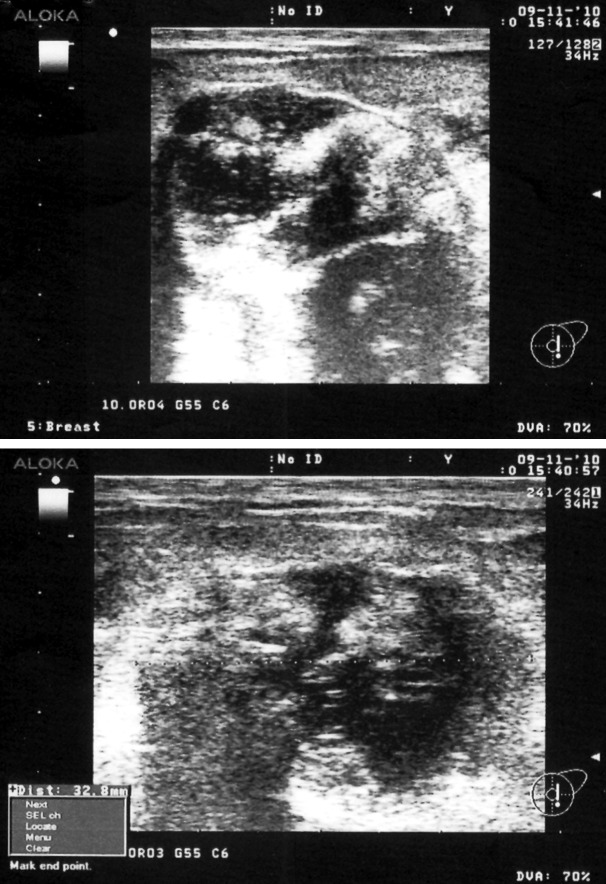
Ultrasound image of primary breast osteosarcoma.
